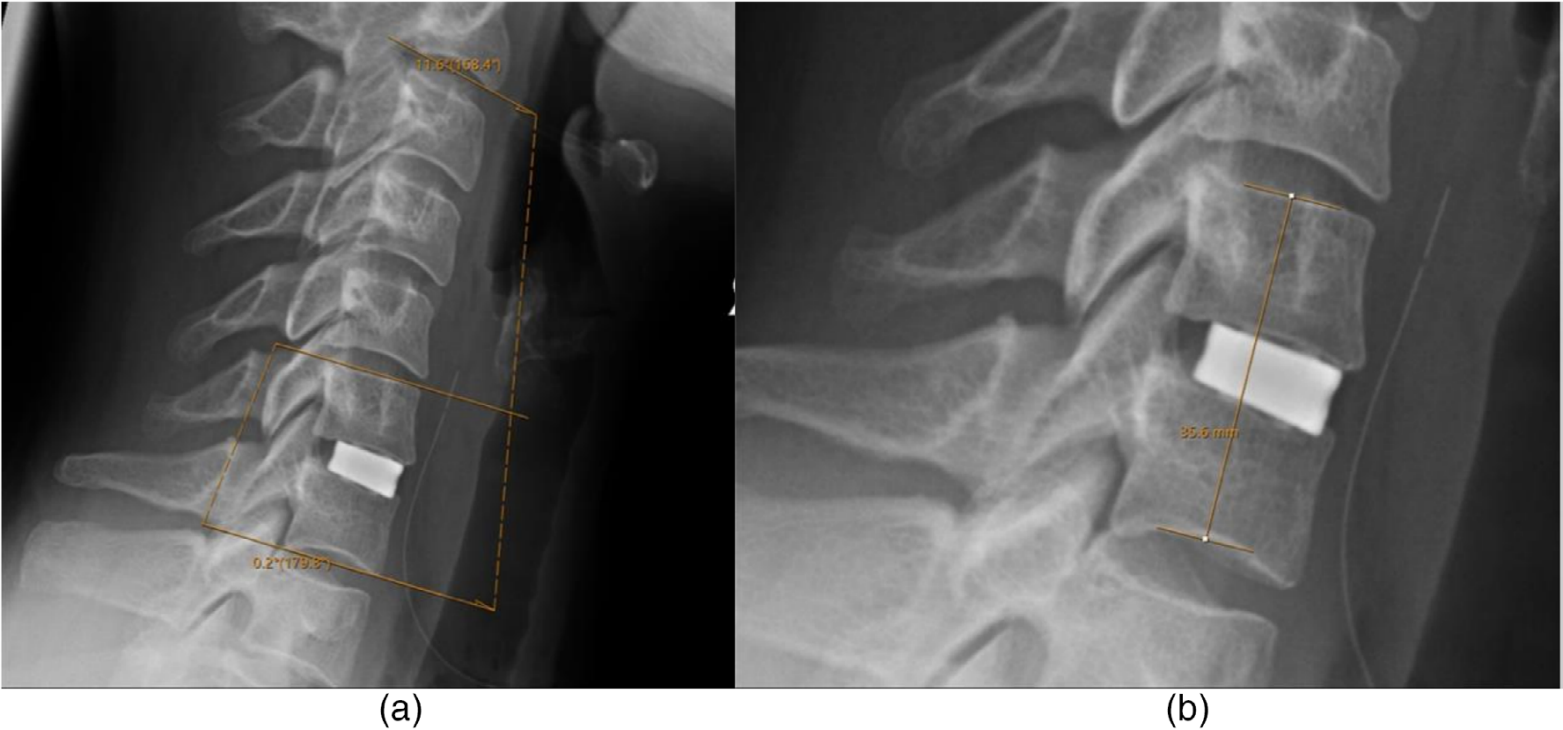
Cervical C2–7 and segmental cobb angle measurements (a) and segmental height measurement across the fused level (b) from neutral lateral radiographs.
